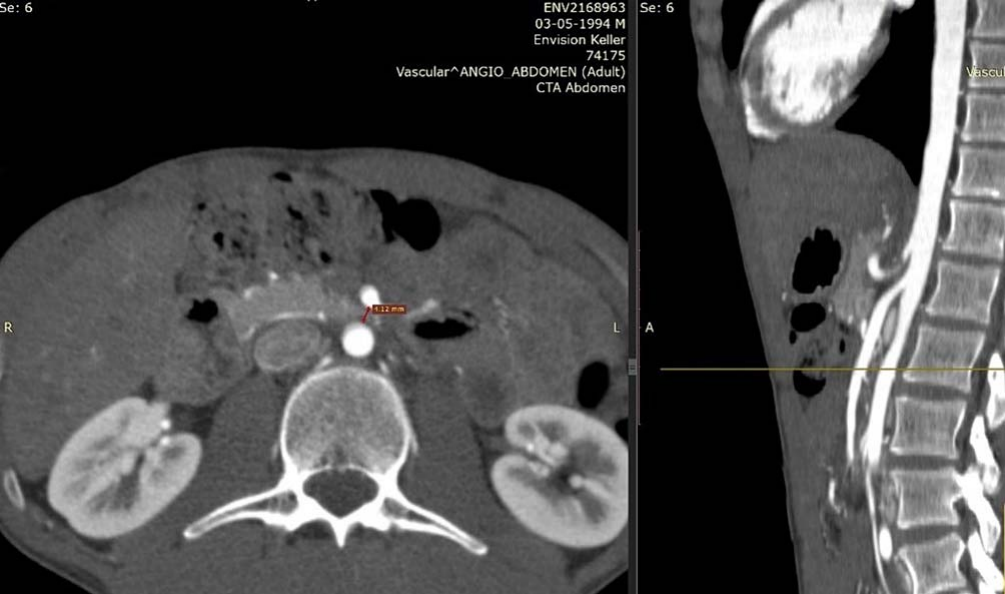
Computed tomography. Aortomesenteric distance of 4 mm. Wilkie’s syndrome.
